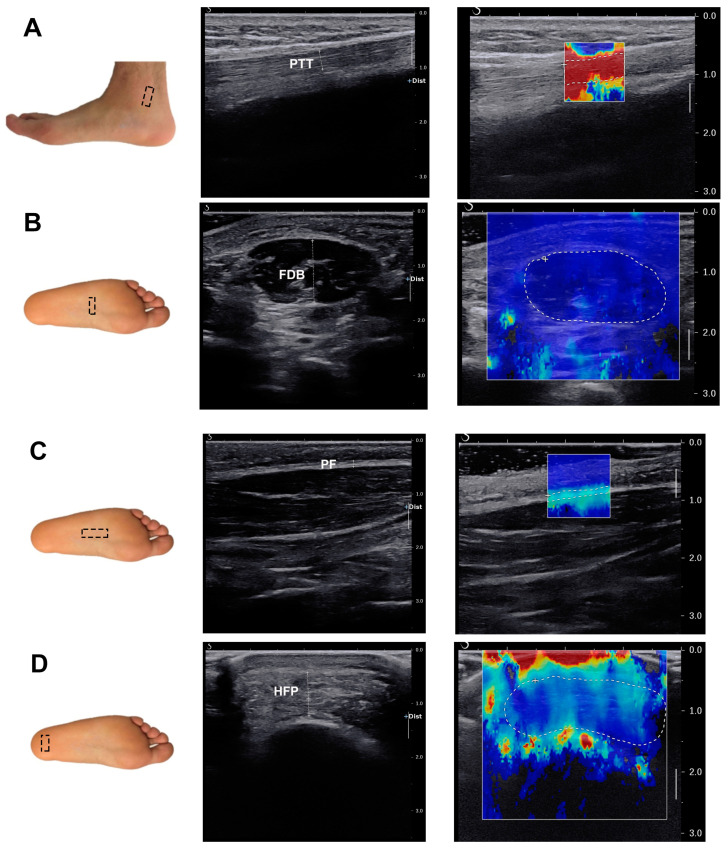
Probe location and ultrasound imaging measurements of PTT (A), FDB (B), PF (C), and HFP (D). The left column shows the locations of probes in the macro views. Dotted boxes represent the locations of the probe. The middle column displays the images of thickness of the four tissues. The right column exhibits the images of elastic modulus of the four tissues. Dashed zones represent ROIs of the four tissues. PTT: posterior tibial tendon; FDB: flexor digitorum brevis; PF: plantar fascia; HFP: heel fat pad.
